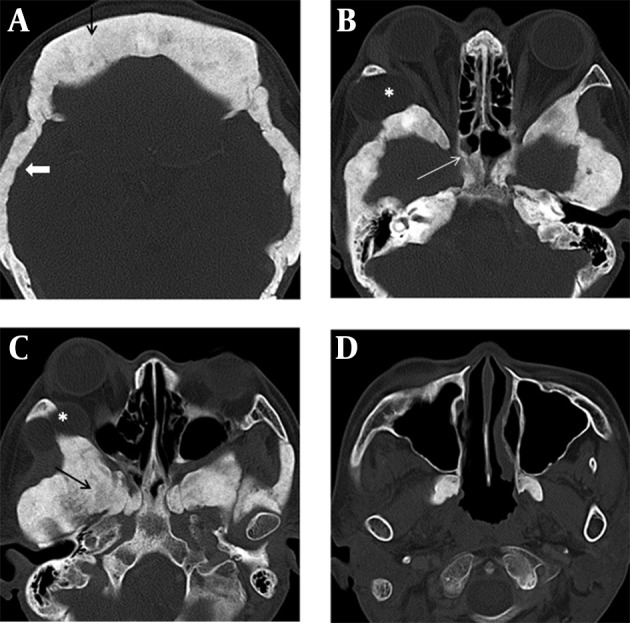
The Maxillary Sinus A, computed tomography of orbit shows hyperostosis of the skull base with complete obliteration of the dipolic space (thick arrow) and frontal sinus (thin arrow). B, the walls of the sphenoid sinuses are thickened and sclerotic (white arrow). C, skull base foramina are encroached (black arrow). D, the maxillary sinus and mandible are spared. B and C, a dermoid cyst at the lateral angle of the right orbit (asterisk).
